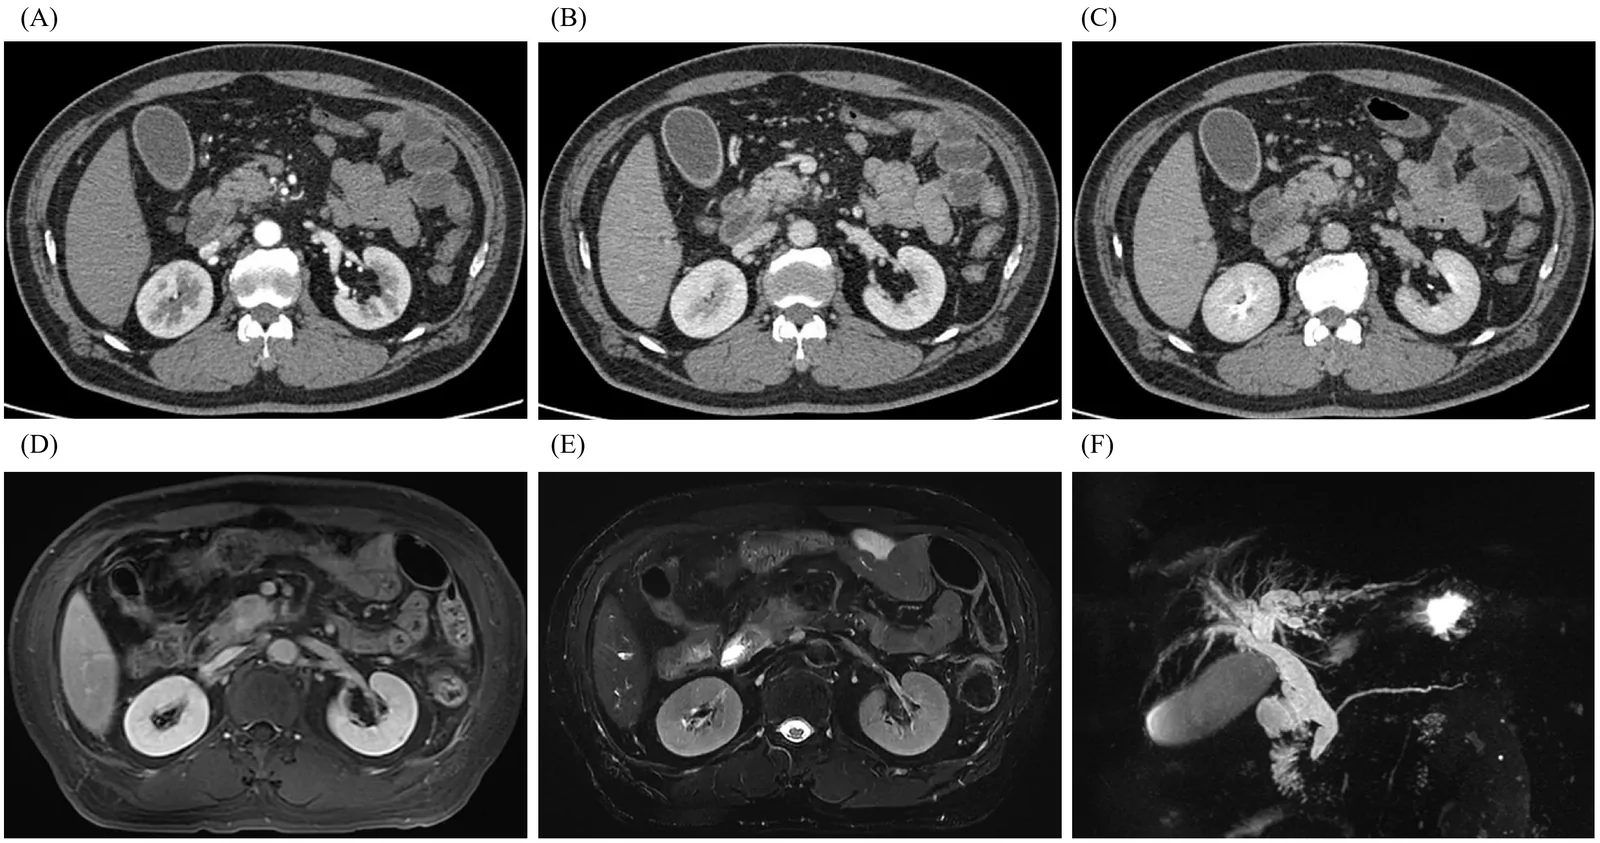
Preoperative abdominal imaging findings. (A–C) Contrast-enhanced CT images show an enhancing nodular lesion in the pancreatic head, which involves the intramural segment of the common bile duct. (D, E) Contrast-enhanced abdominal MRI images show a nodular lesion in the pancreatic head involving the intramural segment of the common bile duct. The lesion shows iso- to slight hypointensity on T1-weighted images and isointensity on T2-weighted images, with mild persistent enhancement on post-contrast sequences. (F) MRCP shows the classic “double duct sign”, with abrupt ductal obstruction at the level of the tumor.(CT, computed tomography; MRI, magnetic resonance imaging; MRCP, magnetic resonance cholangiopancreatography).
